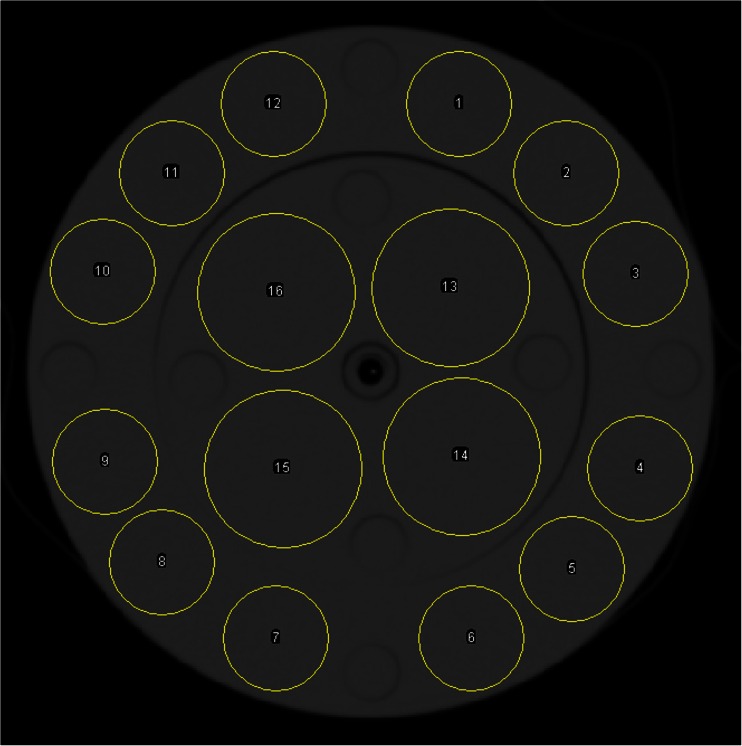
Regions of interest for noise measurements in CT
